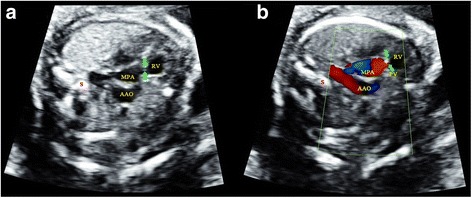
Sonographic images of a 24-gestational-week fetus diagnosed with pulmonary valve atresia. Thickened pulmonary valves (indicated by arrows) were visualized in the right outflow tract view (a) via 2D. However, this anomaly was neglected by the sonographers under Protocol A because the valvular movement was difficult to visualize at times. However, color Doppler resolved this problem by visualizing the retrograde flow in the MPA (b), indicating pulmonary valve atresia. At the same time, no blood flow was detected entering the MPA across the thickened PV. AAO, ascending aorta; MPA, main pulmonary artery; PV, pulmonary valve; RV, right ventricle; S, spine
